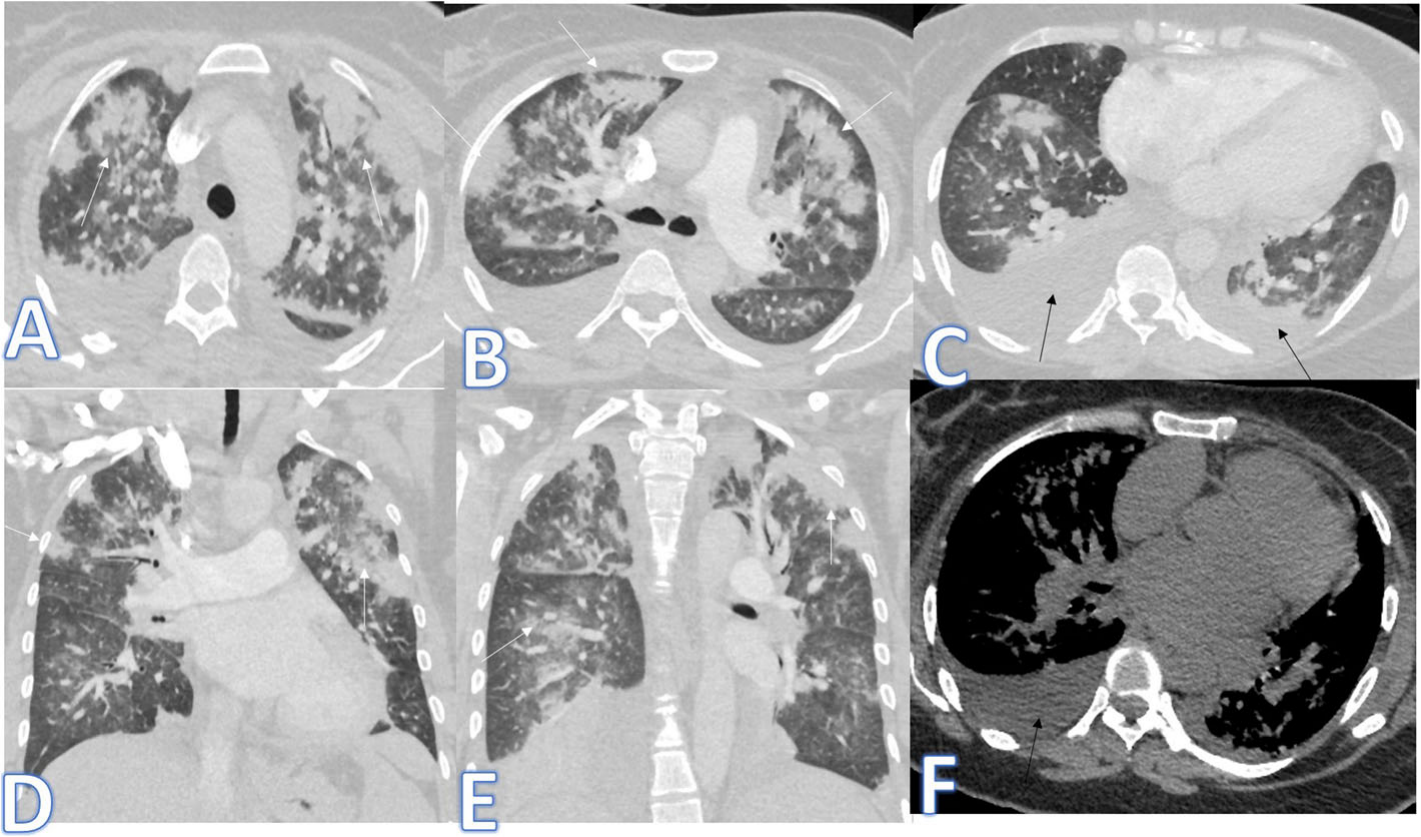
A 49-year-old female patient presented with fever, cough, and dyspnea. CT showed bilateral confluent consolidative patches with upper lobe predilection (white arrows) and bilateral mild pleural effusions (black arrows); the diagnosis was drug rash with eosinophilia and systemic symptoms (DRESS)
